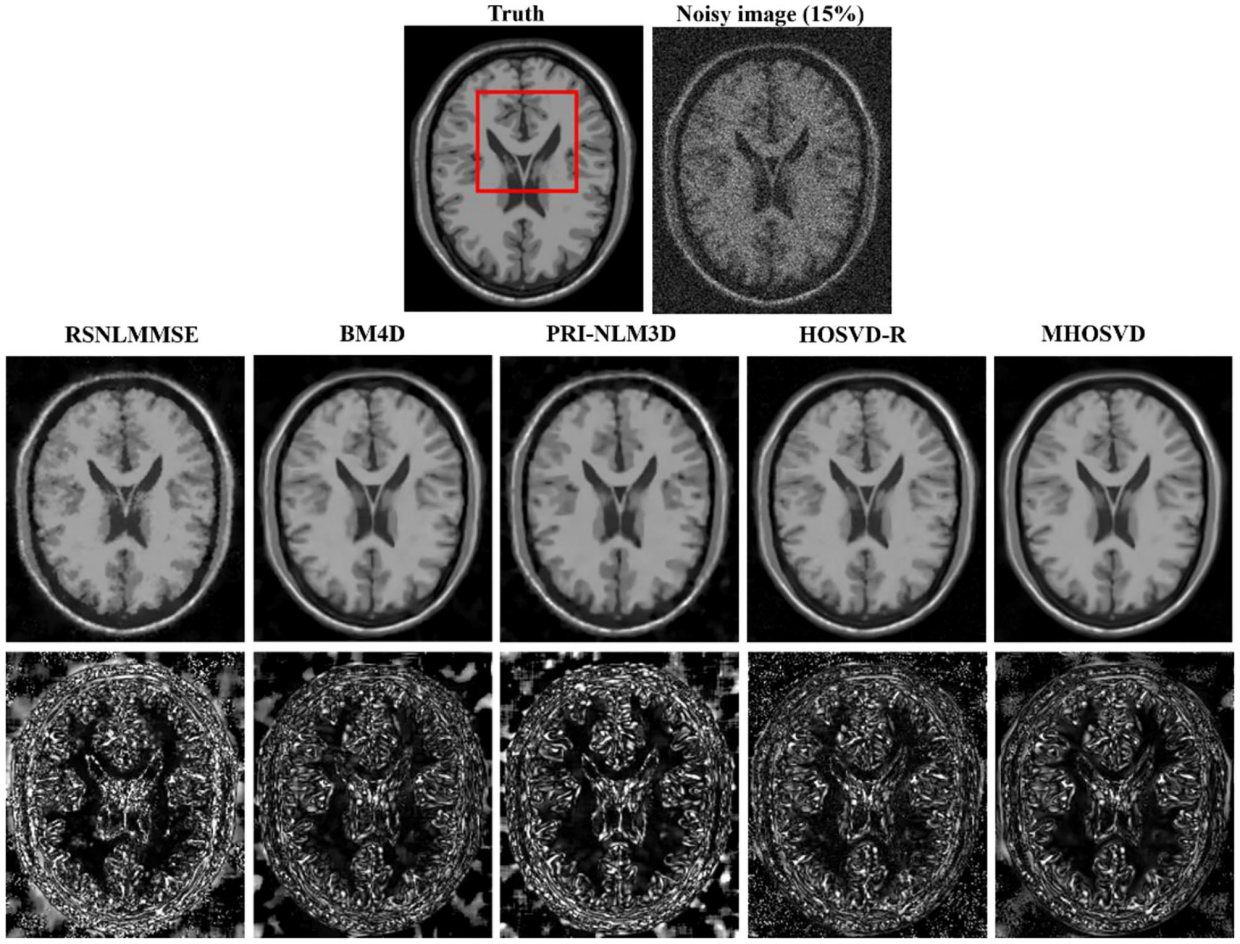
Denoising T1-weighted (T1w) images by different methods (RSNLMMSE, BM4D, PRI-NLM3D, HOSVD-R, and *MHOSVD*) under 15% Rician noise. First line: T2w image without noise and with 15% Rician noise. Second line: the denoised images with four different methods. Third line: the corresponding residual images (the absolute difference between the denoised and noise-free images).
